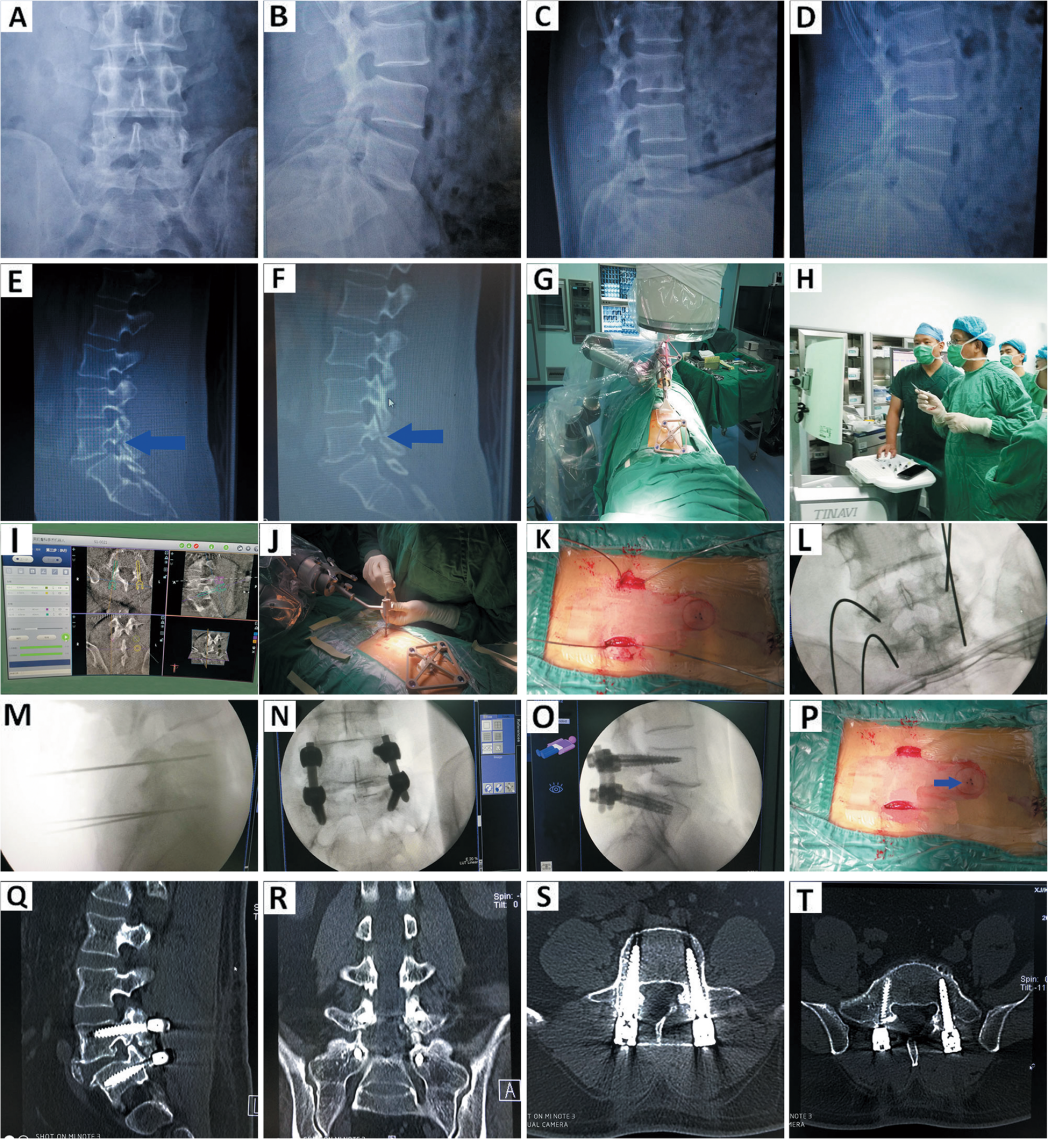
Robot-assisted pedicle screw placement and postoperative image verification with improved minimally invasive tracer fixation. The Patient was diagnosed with L5 lumbar spondylolysis and Ilumbar spondylolisthesis: a-d. preoperative anteroposterior, lateral and flexion-extension radiographs. e-f. preoperative sagittal CT: L5 lumbar spondylolysis and I^。^lumbar spondylolisthesis were marked with arrow(→); g. Three-dimensional image acquisition and registration: the method of improved tracer minimally invasive fixation was display. i. parameter design and path planning of pedicle screw. j-k. guide wire placement. l-m. The position verification of guide wire. n-o. Pedicle screw placement and position verification again. p. Postoperative wound: Minimally invasive wound was marked with arrow(→). q-t. The position grade of pedicle screws were evaluated as A via postoperative 320 CT scan
